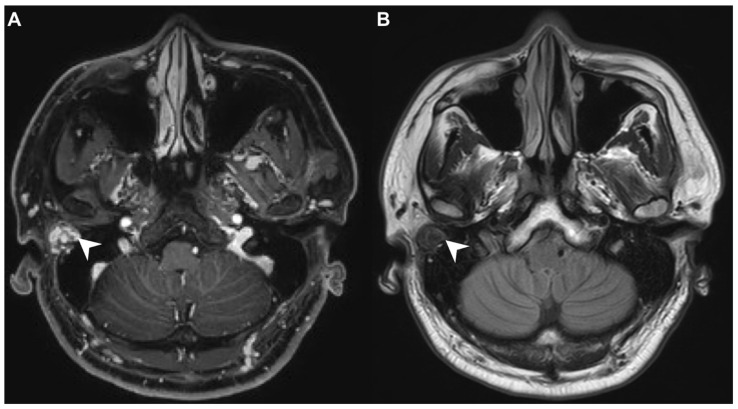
(A) On magnetic resonance imaging (MRI), the T1-contrast sequence revealed a heterogeneous hyperintense mass in the right EAC (arrow). (B) On MRI, the T2-sequence yielded a well-circumscribed isointense signal from the mass (arrow).
